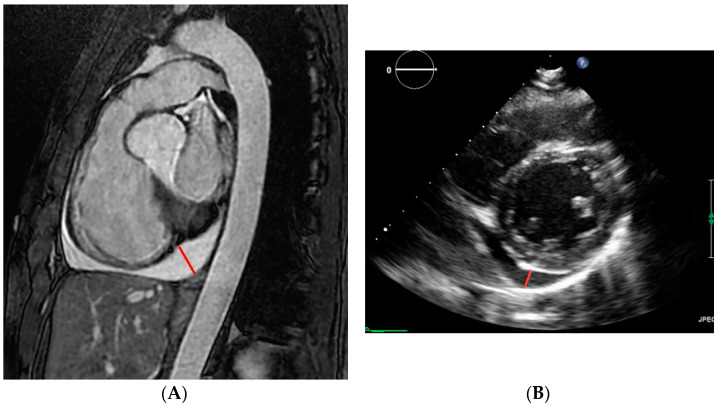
Example of pericardial effusion measurement in ADPKD subjects on (A) MRI and (B) echocardiography. The measurement of pericardial effusion thickness at end diastole is indicated by the red lines.
